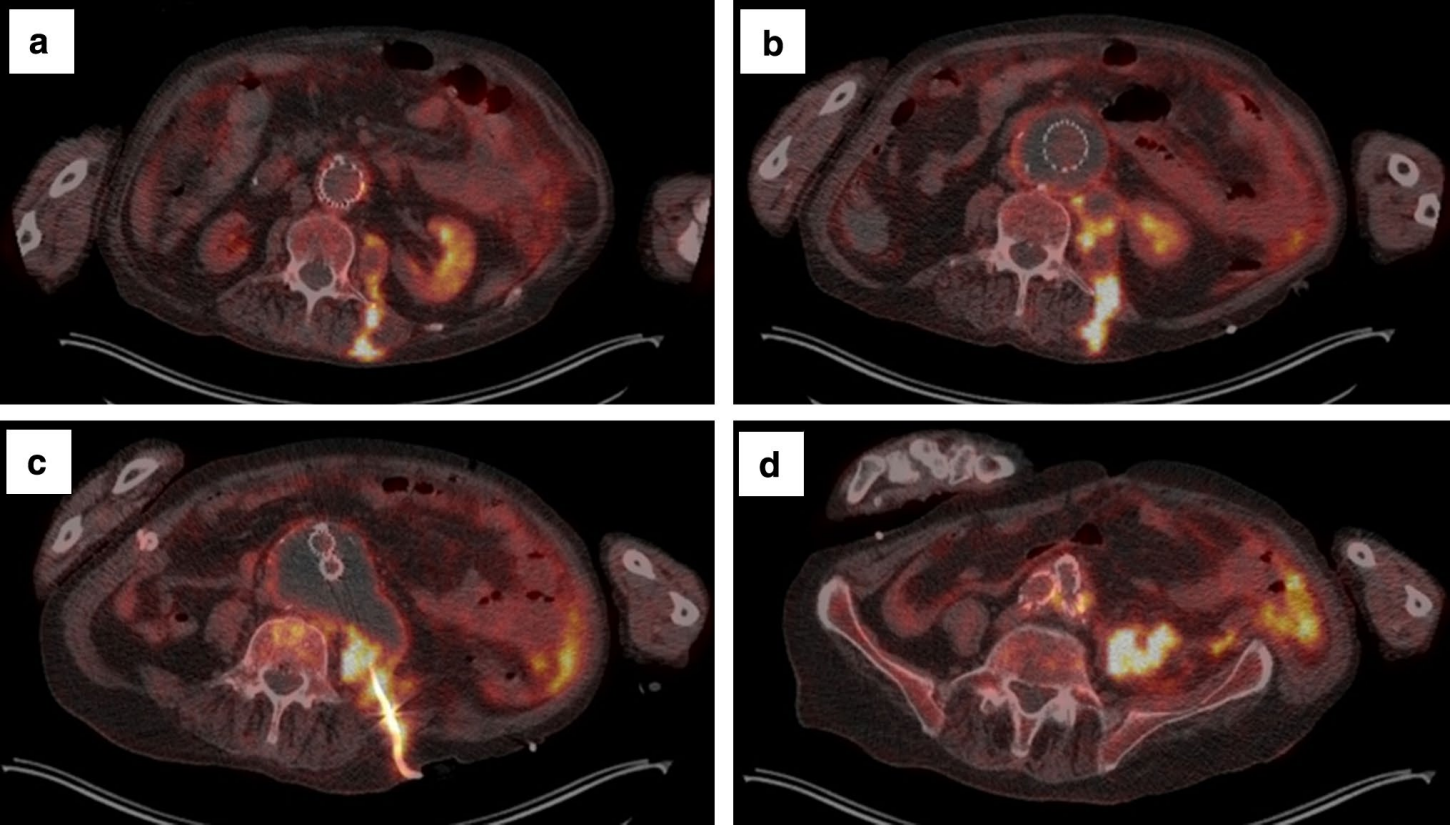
Transversal integrated ^18^F-fluorodeoxyglucose positron emission tomography/low-dose CT (^18^FDG-PET/CT) images, from cranial to caudal, demonstrating: a increased FDG-uptake in the left iliopsoas muscle dorsally extending through the musculature of the back, and increased FDG-uptake in the wall of the aortic aneurysm adjacent to the endovascular aneurysm repair (*EVAR*). b A per continuitatem infection arising from the abdominal aortic aneurysm (*AAA*), thrombosis of aortic aneurysm and low activity in the cavity of the EVAR resulting from blood flow. The infection extends to the abscess and left iliopsoas muscle. c Percutanous drain in situ in the abscess, increased FDG-uptake in the cranial portion of the vertebra, and increased FDG-uptake in adipose tissue of the left abdominal wall in continuitatem with the abscess (not visible at the level of this transversal slice). d Increased FDG-uptake in the aortic wall adjacent to the caudal part of the EVAR, and increased FDG-uptake extending into adipose tissue of the left abdominal wall
